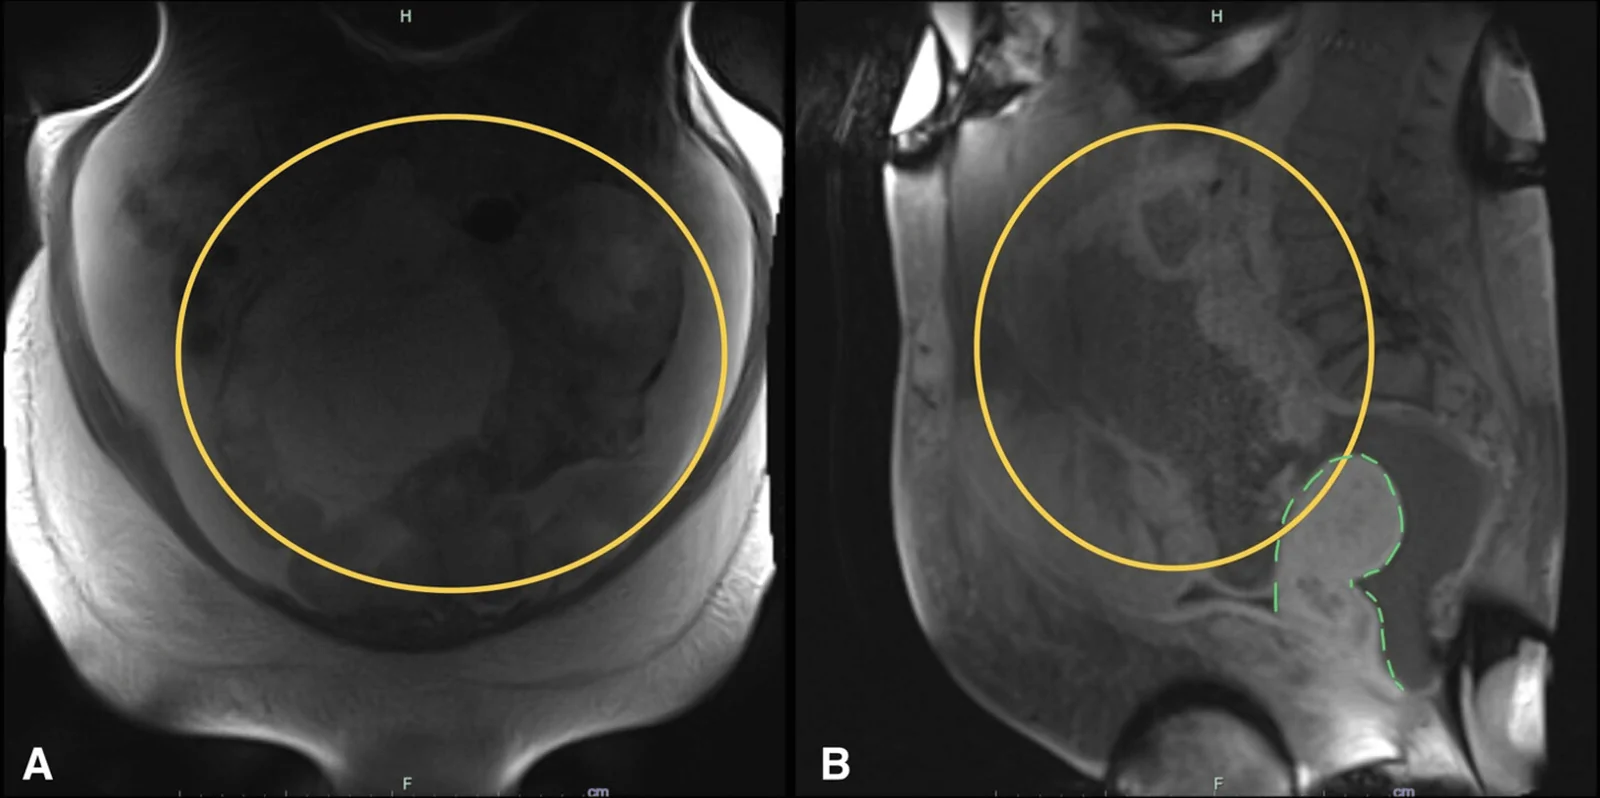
Pelvic magnetic resonance imaging (MRI) Demonstrates a large heterogeneous adnexal mass with cystic and solid components. (A) Coronal T2-weighted image shows the lesion (yellow circle) with hyperintense cystic areas and mild ascites. (B) Sagittal T1-weighted image confirms the large heterogeneous mass (yellow circle), with the uterus outlined by the green dashed line.
